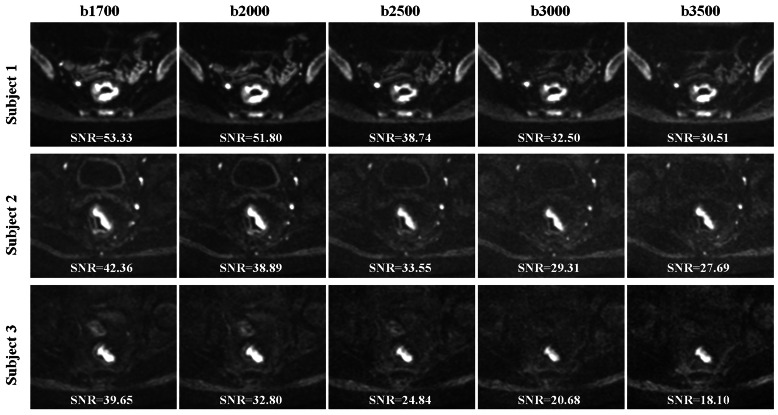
The tumor SNR at ultra-high b-value DWIs. The subject 1 (female, 26-year-old, TNM-stage III) represents highest SNR of first ten multi-b value DWI scans, subject 2 (male, 47-year-old, TNM-stage III) represents median SNR and subject 3 (male, 51-year-old, TNM-stage II) represents lowest SNR. Even with the lowest SNR, the tumor still could be clearly identified on b3500 DWI image
